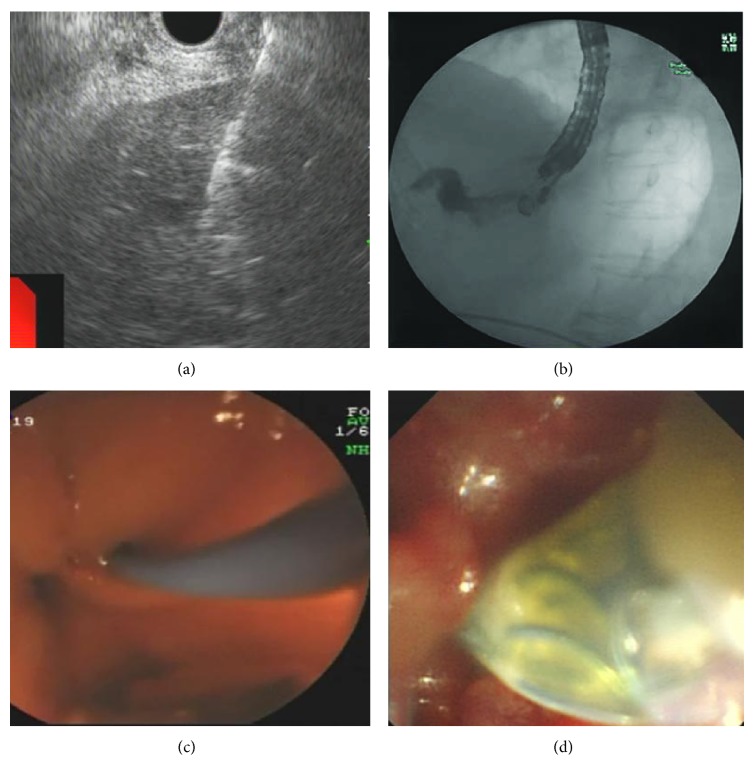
Endoscopic ultrasound-guided hepaticogastrostomy. Under real-time EUS guidance, the dilated intrahepatic bile duct was punctured and a cholangiogram was obtained (a and b). After transmural fistula dilation (c), a fully covered self-expandable metal stent was deployed (d).
